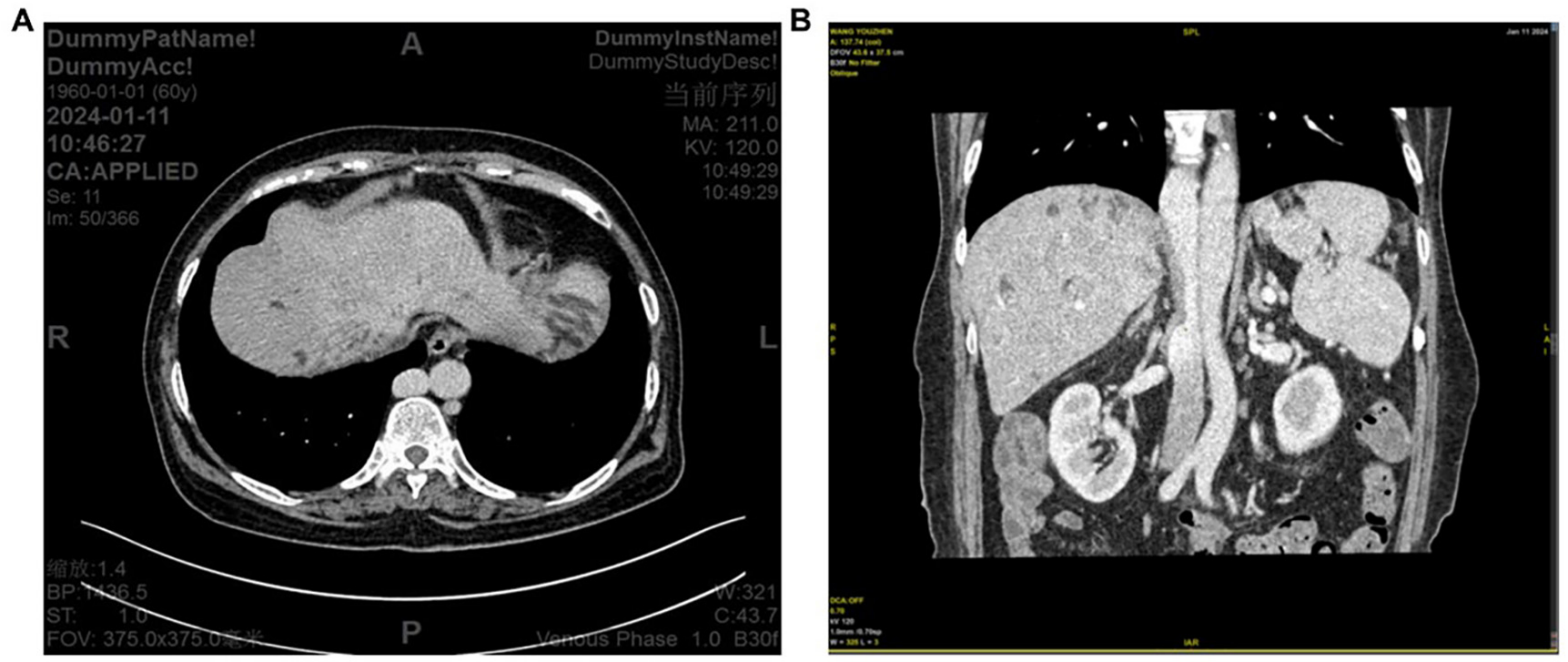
(A) Computed tomography (CT) image showing azygos vein dilation and tortuosity. (B) CT image showing focal non-visualization of the inferior vena cava (IVC).
